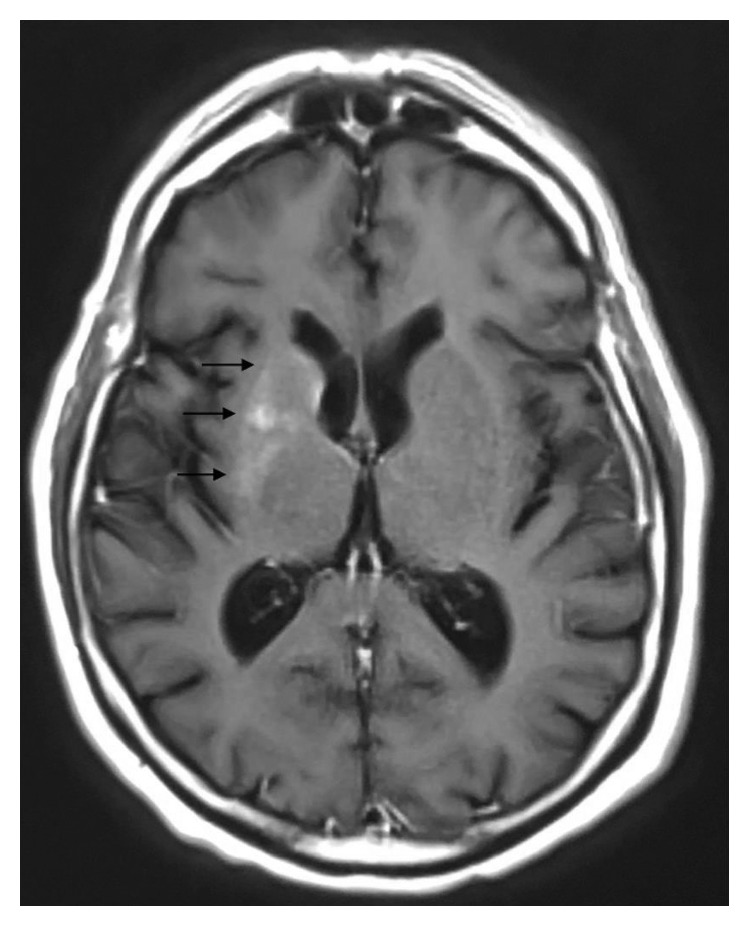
MRI brain postcontrast T1-weighted axial spin echo image. Arrows highlight hyperintense signal abnormalities in the right basal ganglia with no change in enhancement postcontrast.
